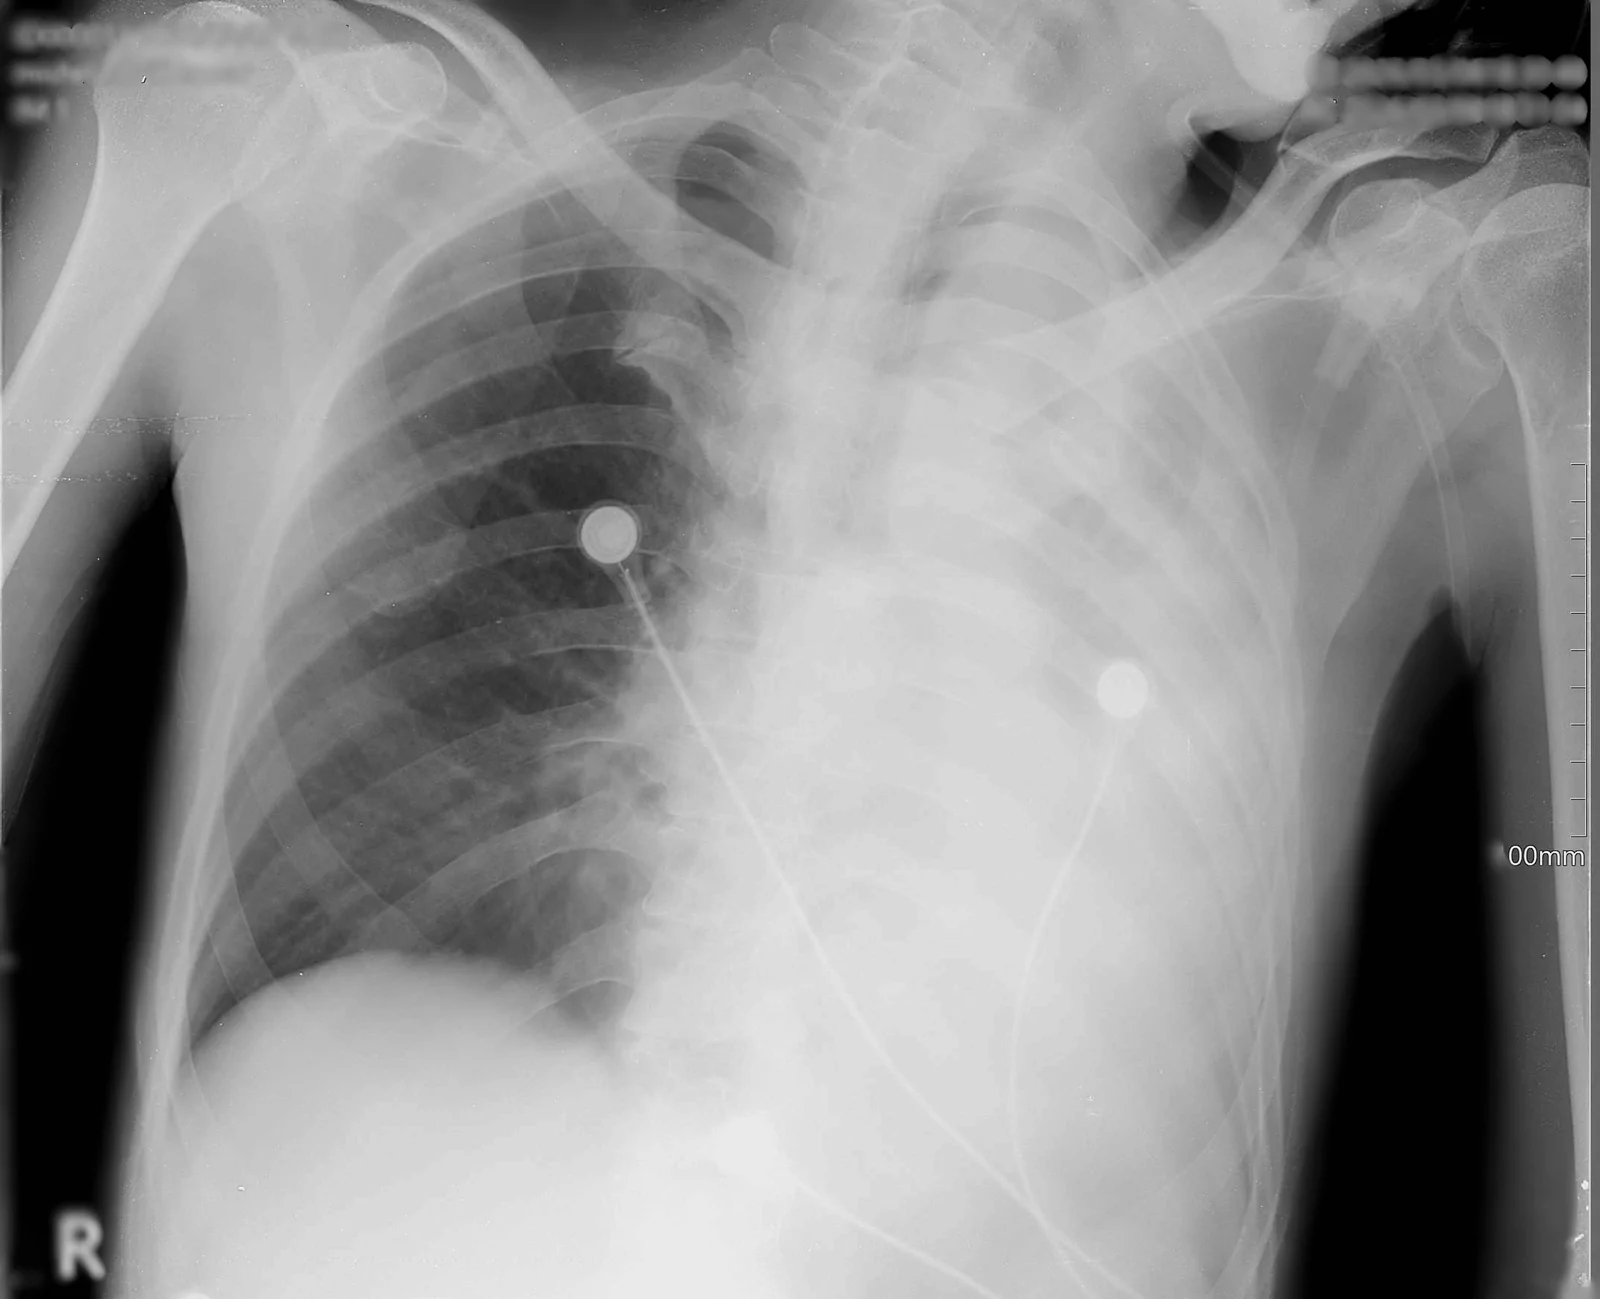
Portable anteroposterior chest radiograph obtained during the terminal respiratory course demonstrating near-complete opacification of the left hemithorax due to extensive left-sided air-space consolidation with associated pleural effusion, consistent with severe pneumonia
